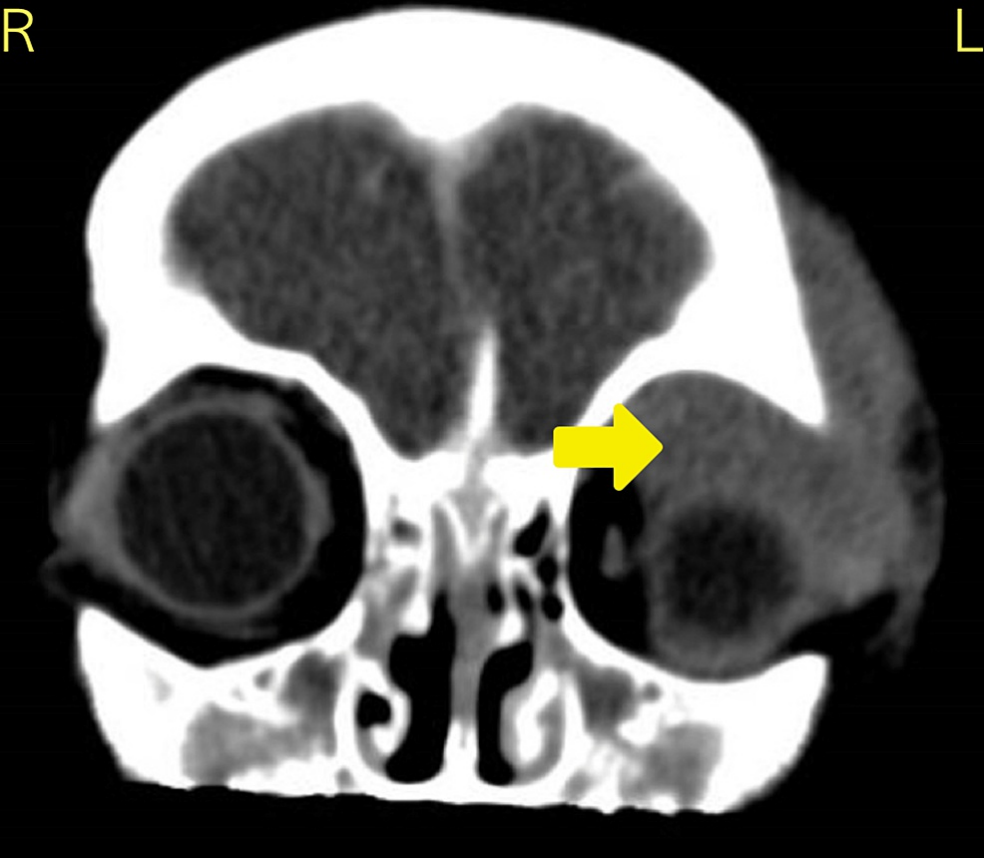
Coronal CT scan of brain and paranasal sinuses for case 1 revealed an aggressive left-sided superior orbital mass (arrow). There is inferior displacement of the globe, poor differentiation of superior and lateral recti muscles, and significant invasion of superotemporal soft tissue.
